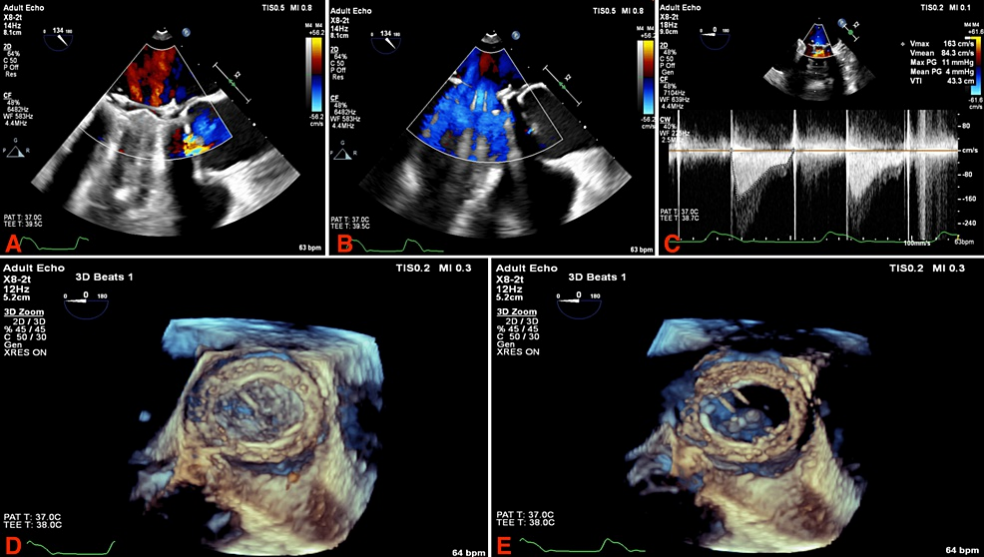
Intra-operative transesophageal echocardiogram. (A) 2D visualization of the mitral prosthesis during systole with color doppler; (B) 2D visualization of the mitral prosthesis during diastole with color doppler; (C) pressure gradients across the mitral prosthesis; (D) 3D visualization of the mitral prosthesis during systole; (E) 3D visualization of the mitral prosthesis during diastole.
